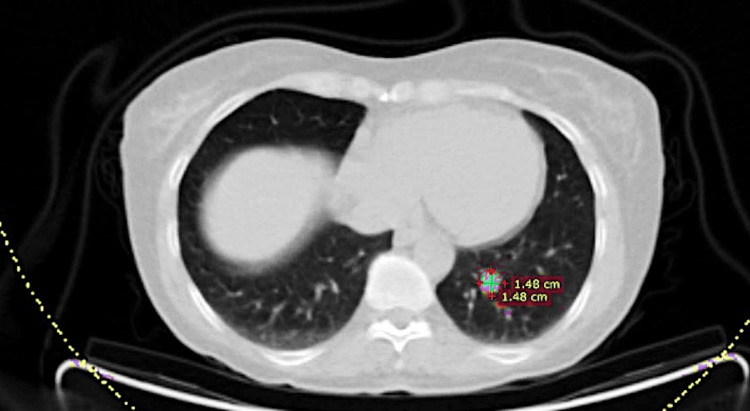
Axial view of the CT scan showing the SFT in the left lower lobe in 2010 CT: computed tomography; SFT: solitary fibrous tumor
